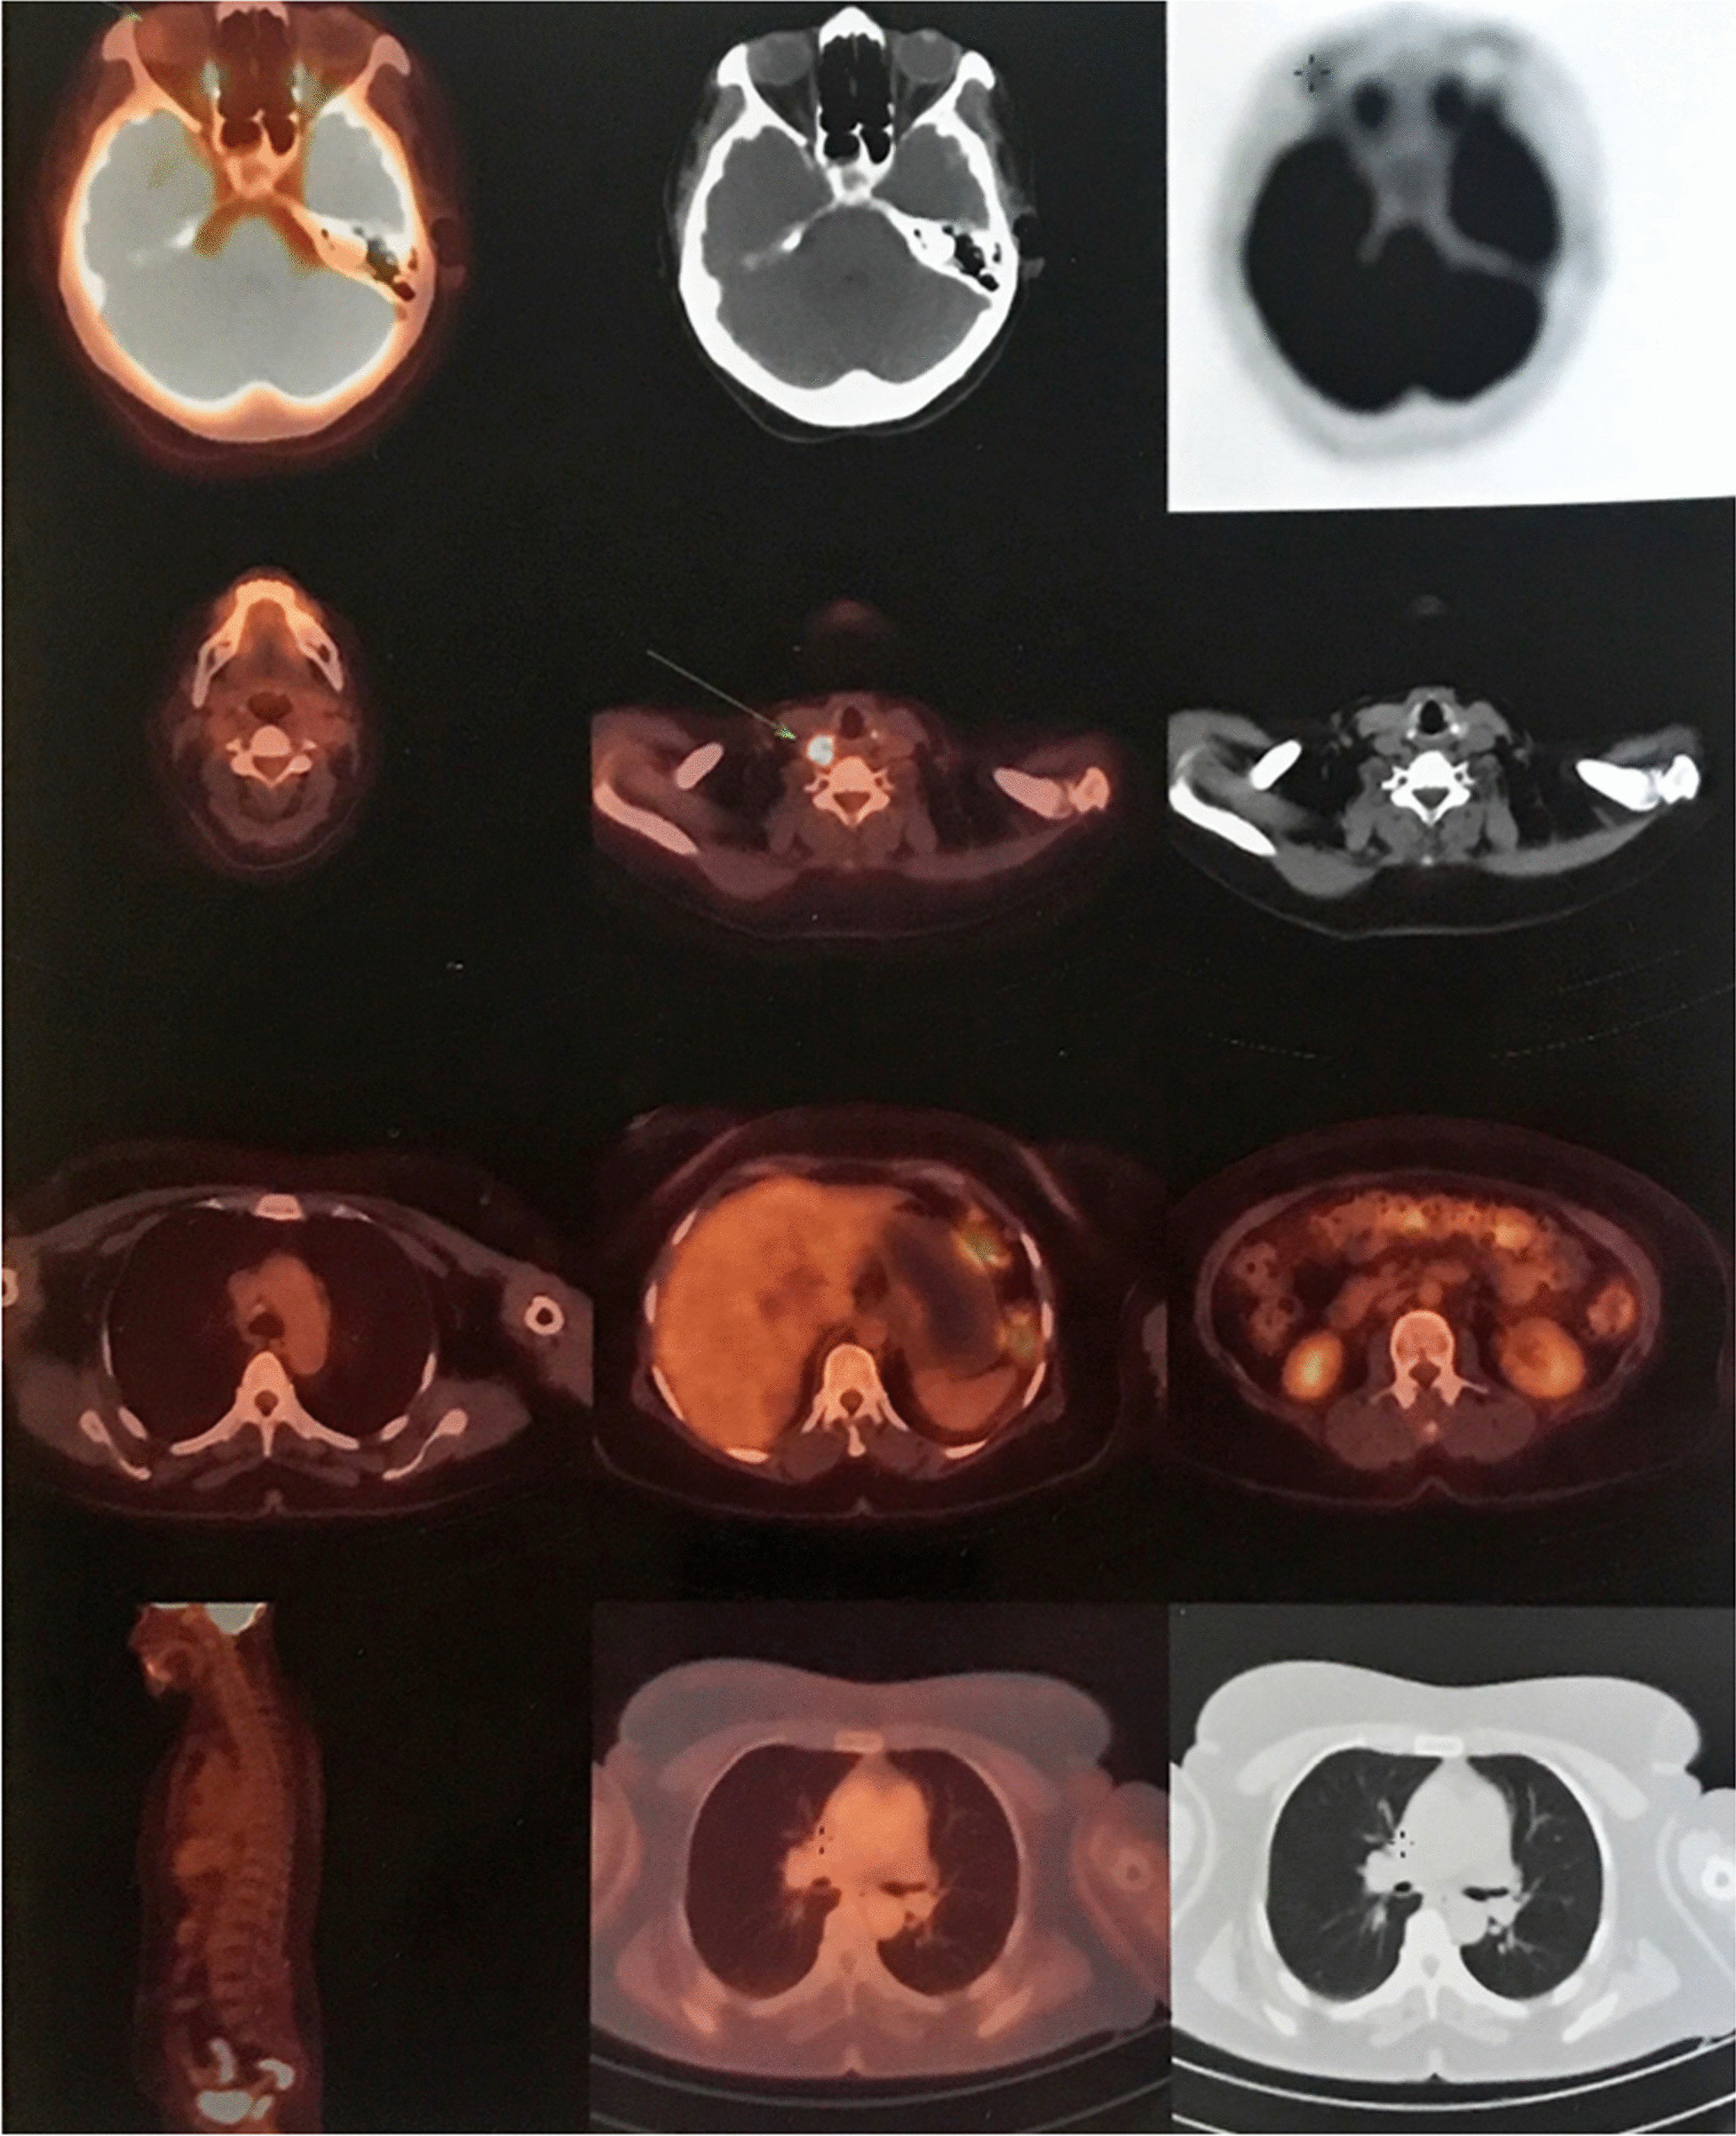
FDG-PET/CT scan: low-grade metabolically active tumoral involvement in the anterolateral aspect of the right lobe of thyroid as well as hypermetabolic lymphadenopathy at cervical zone VI
